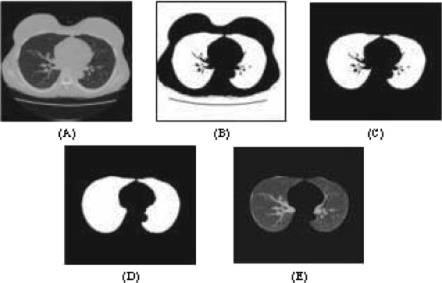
(Color) Automatic extraction of the lungs from chest CT images. (*A*) Original image. (b) Thresholded image. Threshold level=−375 HU. (c) Initial lung mask. (d) Complete lung mask. (e) Extracted lungs.
